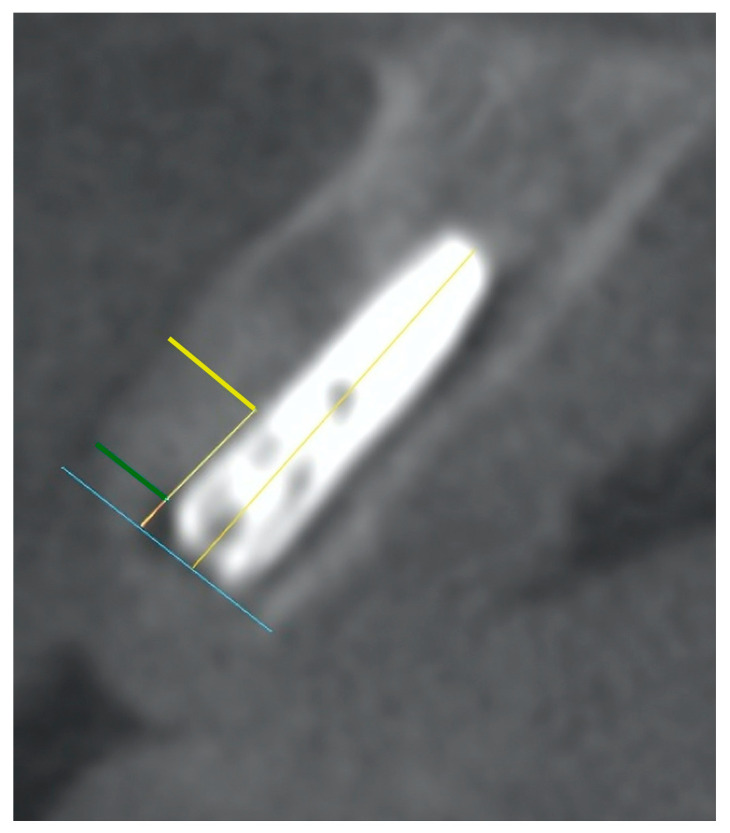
CBCT measurements and landmarks: blue line = implant collar reference; green line = R1, which is located 1 mm apical to the implant collar reference; yellow line = R2, which is located 5 mm apical to the implant collar reference.
